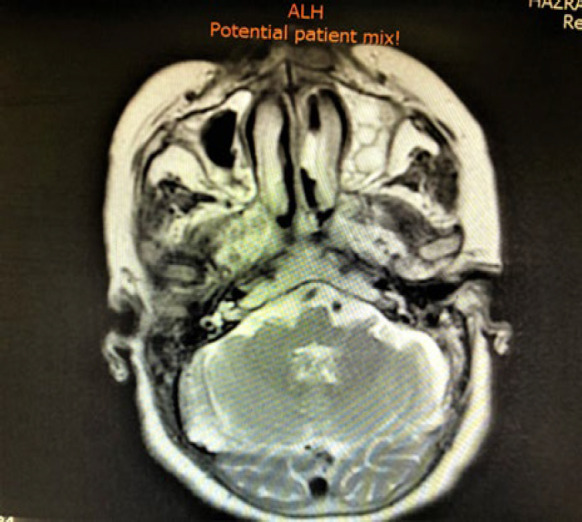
MRI imaging shows the primary mass in the nasal cavity, extended to the nasal bone with foci of metastasis in the brain
